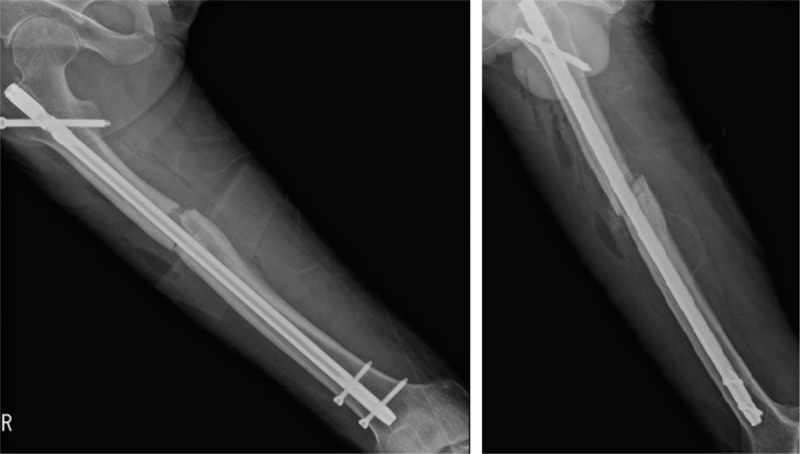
Radiographs after exchanging nailing using a new nail.
